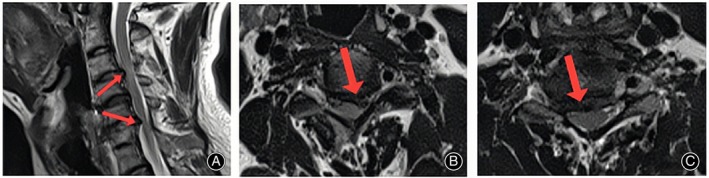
Preoperative T2‐weighted MRI scan. sagittal position showed that the spinal cord was obviously compressed by the C3/4 and C5/6 level intervertebral disc‐osteophyte complex (A), and the transverse position showed that the C3/4 level (B) and C5/6 level (C) intervertebral disc‐osteophyte complex compressed the ventral spinal cord.
